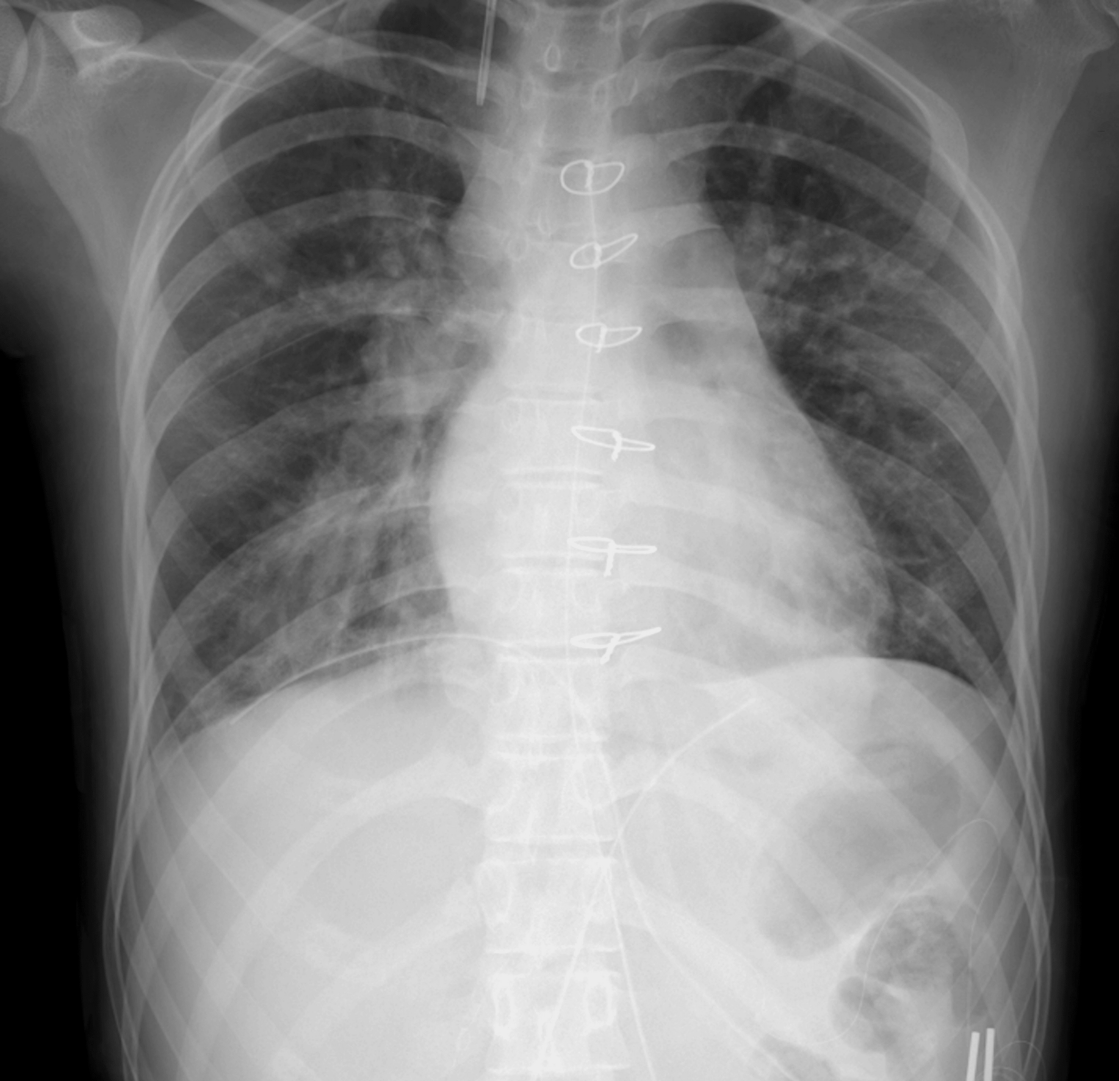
Chest radiograph obtained before extubation
